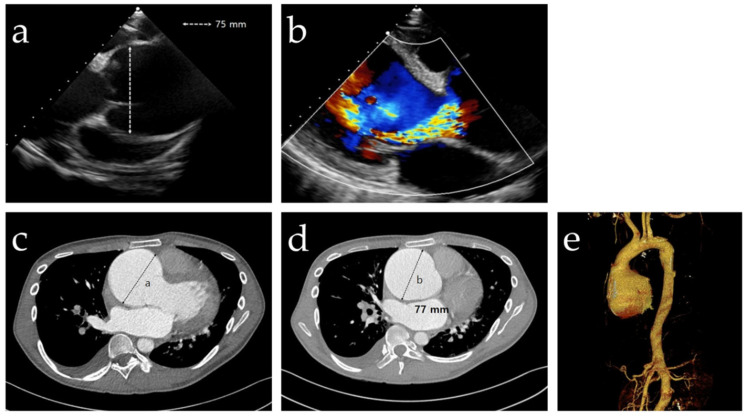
Radiologic findings of the proband. (a,b) Transthoracic echocardiography revealed a valsalva sinus aneurysm (dotted arrow) with severe aortic valve regurgitation. (c,d) The computed tomographic aortogram (CTA) showed a valsalva sinus aneurysm and proximal ascending aortic aneurysm in the trans-sectional images. (e) The three-dimensional reconstructed image of the CTA revealed annuloaortic ectasia.
